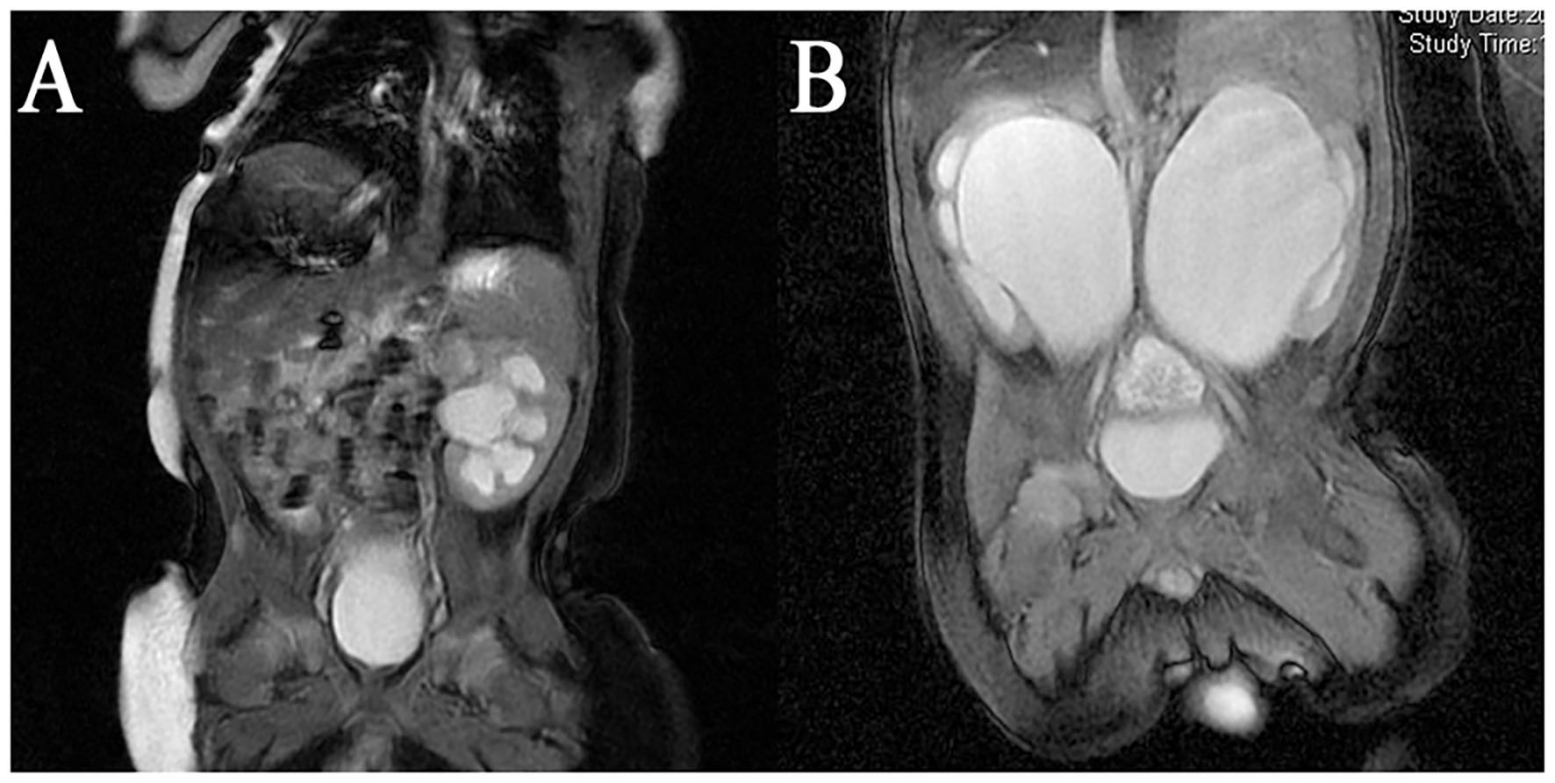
MRI result of a (A) Unilateral UPJO with contralateral renal dysplasia; (B) bilateral severe UPJO.
